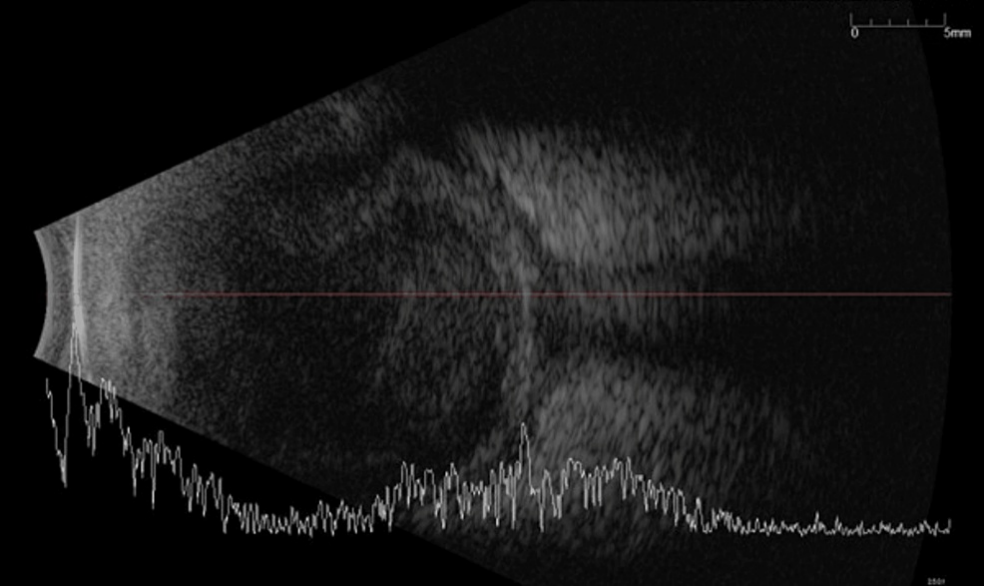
Ultrasound B-scan showing dense vitreous loculation and diffuse scleral thickening (T-sign).
